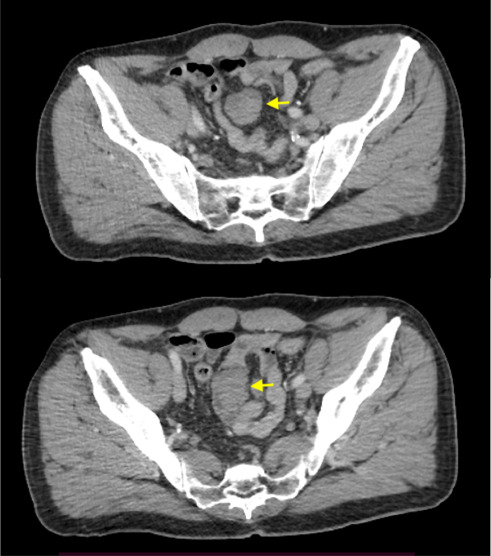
CT scan with intravenous contrast revealed a well-defined mesenteric mass measuring 3.5 × 3.2 cm in the pelvic region (yellow arrow).
